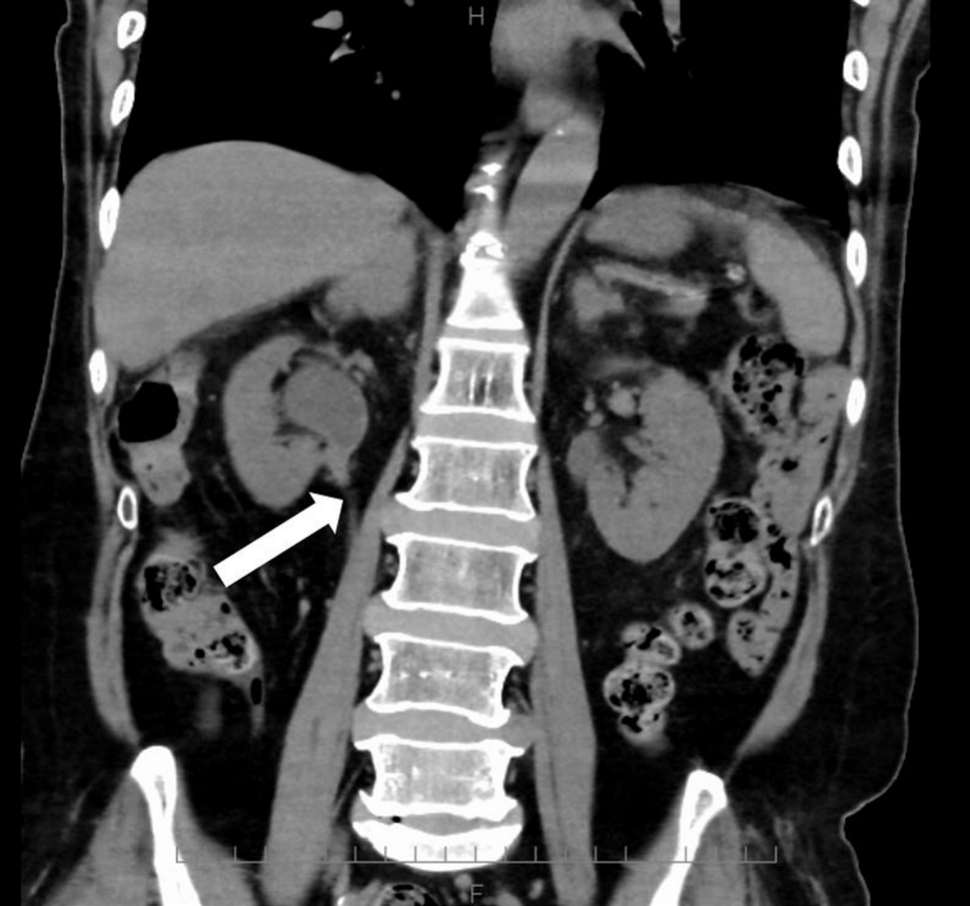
Computed tomography image on day 0. Origin of obstruction in the right ureteral transition and dilatation of the right renal pelvis (arrow)
